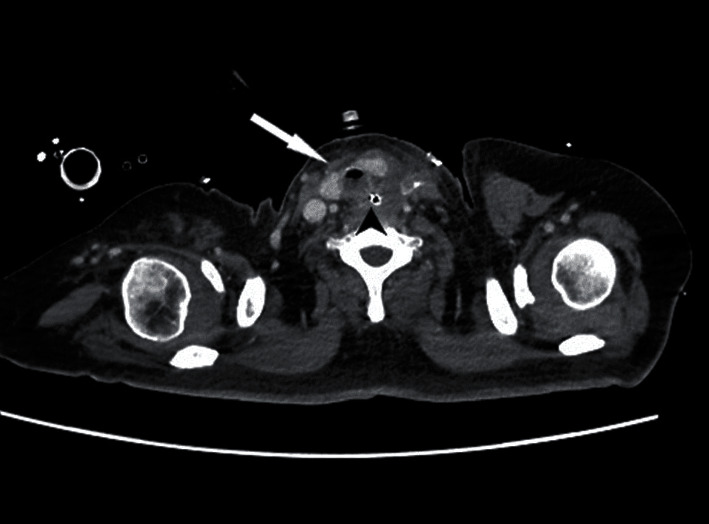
Postoperative neck CT. A neck CT obtained on postoperative day 10 shows no free air between the trachea (arrow) and the esophagus (arrowhead) at the level of the tracheoesophageal fistula repair (above the tracheostomy).
